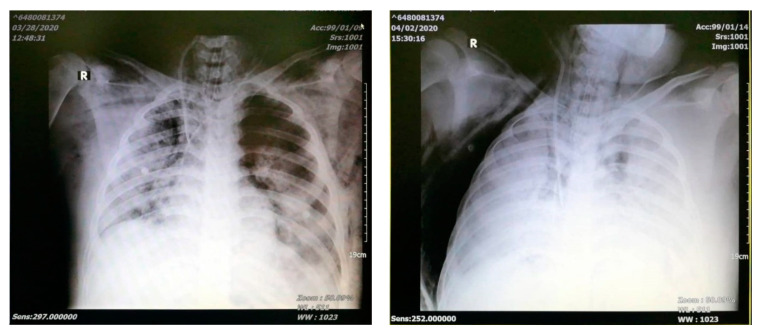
Chest X-ray on day 1 (left) and day 4 (right).
